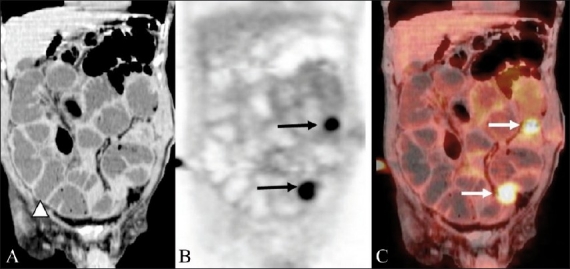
A 52-year-old man treated for CRC presented with abdominal distention and vomiting. Coronal unenhanced CT scan (A) shows multiple dilated small bowel loops (arrowhead). The coronal PET image (B) reveals discrete foci of abnormal FDG uptake in the abdominal cavity (arrows). These foci were mapped to the peritoneal surface of the small bowel (arrow) on the fusion PET / CT image (C), suggesting metastatic peritoneal implants as the cause of intestinal obstruction
